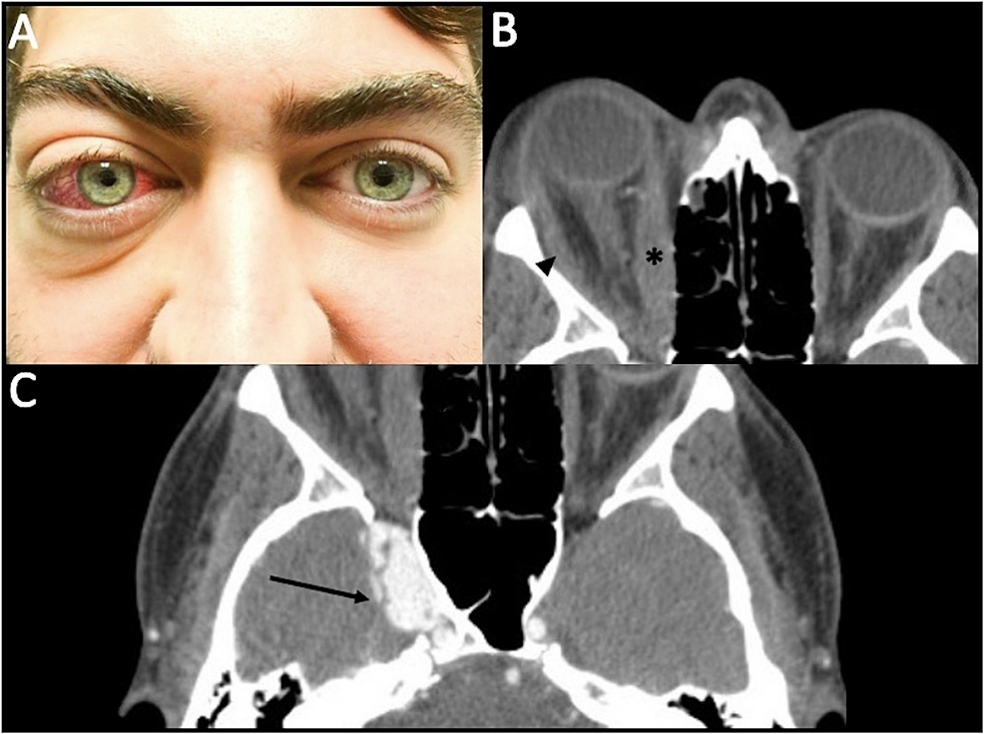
Pre-operative external ocular examination and orbital imaging. (A) External photograph demonstrating proptosis, periorbital edema, and diffuse conjunctival injection of the right eye. (B) Computed tomography scan of the orbits (axial view) demonstrating right-sided proptosis, asymmetric enhancement, as well as enlargement of the right medial (asterisk) and lateral rectus muscles (arrowhead). (C)  Computed tomography scan of the orbits and head showing enhancement of the right cavernous sinus (arrow).
